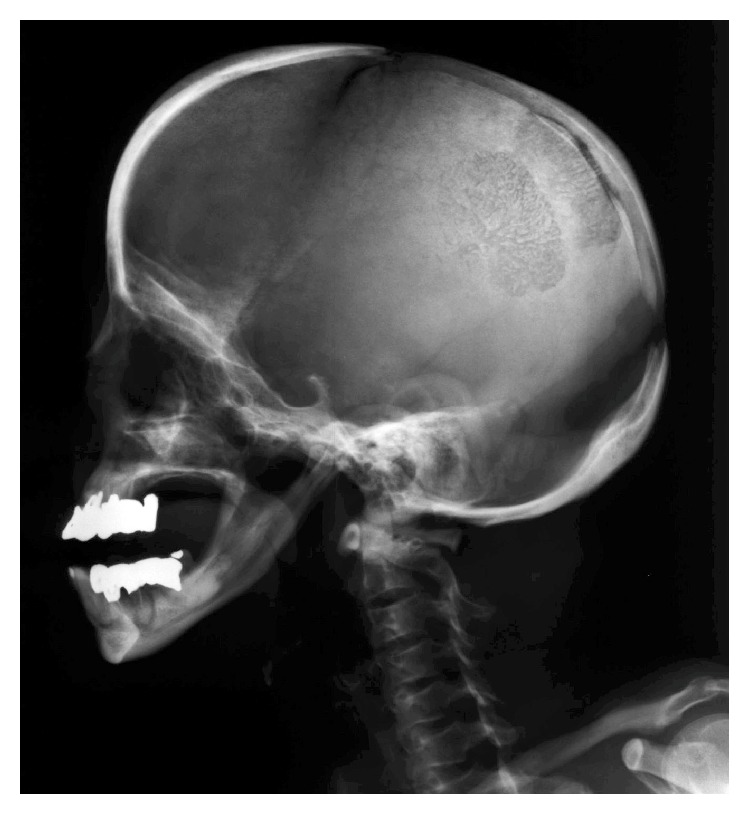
Lateral radiograph of the skull showed separated sutures, open fontanelle, and obtuse angle of mandible.
